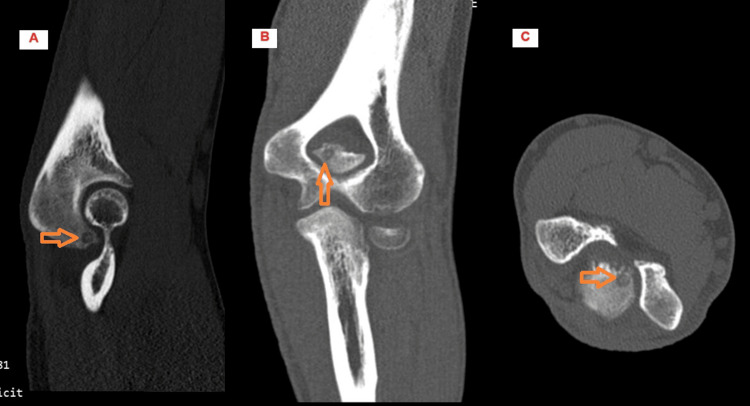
CT Scans of Elbow Sagittal (A), coronal (B), and axial (C) views of osteoid nidus located at olecranon tip (arrows) shown in elbow CT images ten months after onset of symptoms.
